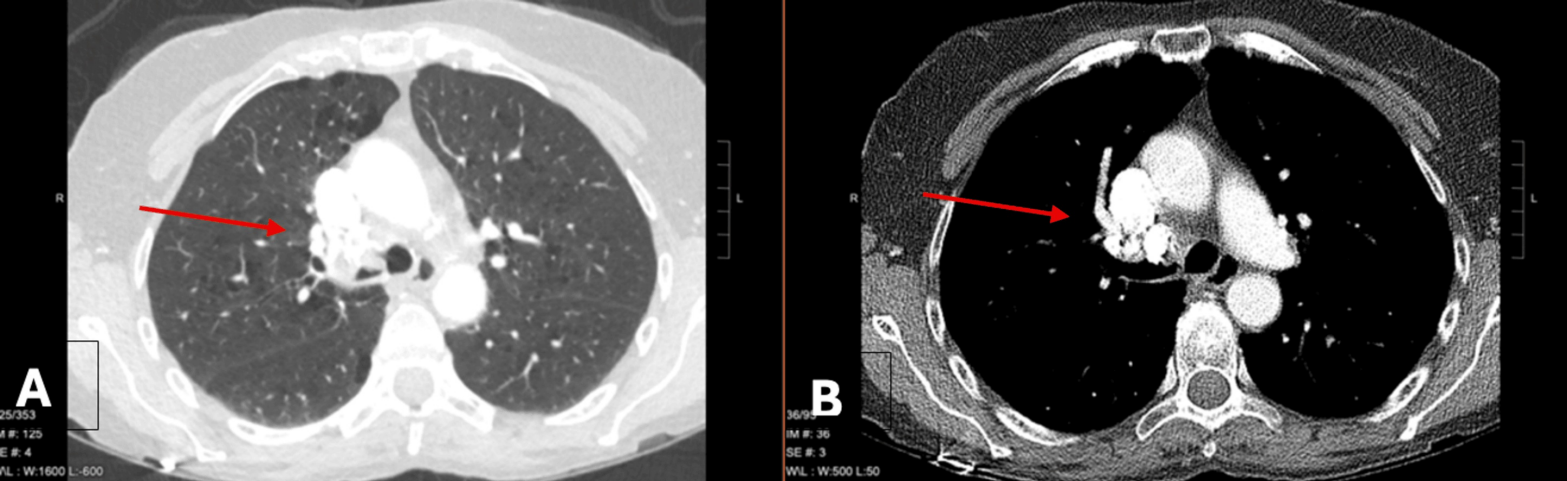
Computed tomography findings of broncholithiasis in Case 1. Axial contrast-enhanced chest CT images displayed in lung window (A) and mediastinal window (B) demonstrate calcified mediastinal lymphadenopathy with a large, densely calcified station 4R lymph node protruding into the right mainstem bronchus, resulting in significant airway narrowing (arrows). These findings are consistent with broncholithiasis secondary to prior granulomatous disease.
